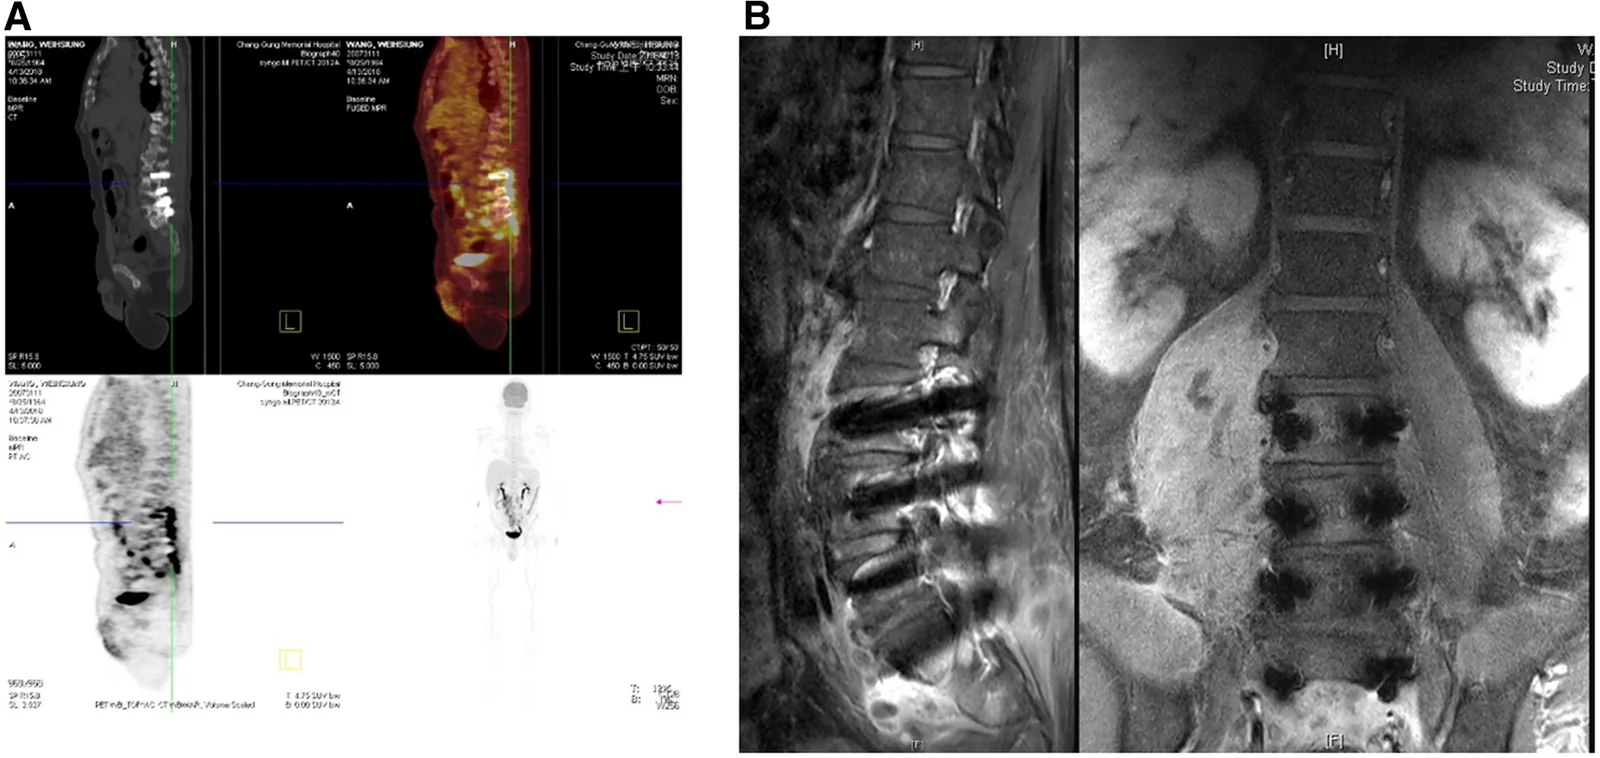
Advanced imaging findings of late peri-prosthetic infection associated with the DDS system. (A) PET/CT longitudinal and coronal reconstructions showing intense, localized radiotracer hypermetabolism surrounding the lumbosacral instrumentation, indicative of active peri-prosthetic infection. (B) Gadolinium-enhanced magnetic resonance imaging (MRI) showing extensive peri-prosthetic inflammatory change with associated paraspinal and deep soft tissue abscess formation around the stabilized lumbar segments. CT = computed tomography, DDS = Dynesys Dynamic Stabilization, MRI = magnetic resonance imaging, PET = positron emission tomography.
